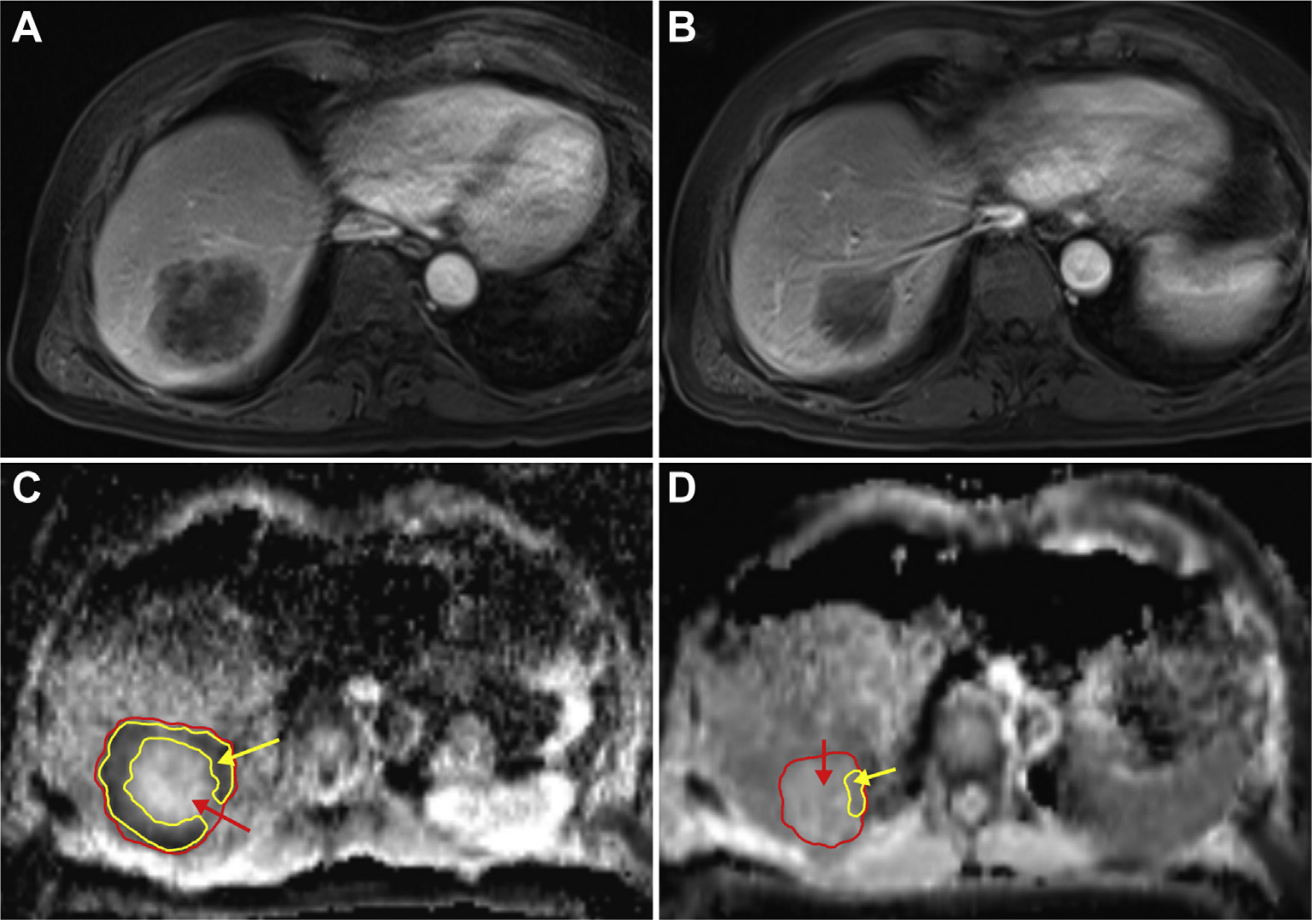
Contrast-enhanced magnetic resonance imaging (MRI) and diffusion-weighted imaging. A gadolinium-enhanced T1 volumetric interpolated breath-hold examination (VIBE) MRI of a liver metastasis before (A) and after (B) chemotherapy. The corresponding apparent diffusion coefficient (ADC) maps calculated from diffusion-weighted images (DWI) of the same tumour before (C) and after systemic therapy (D). Red area and arrow: whole tumour area. Yellow area and arrow: the postulated active tumour area (ADC periphery, the C-formed rim in image C).
